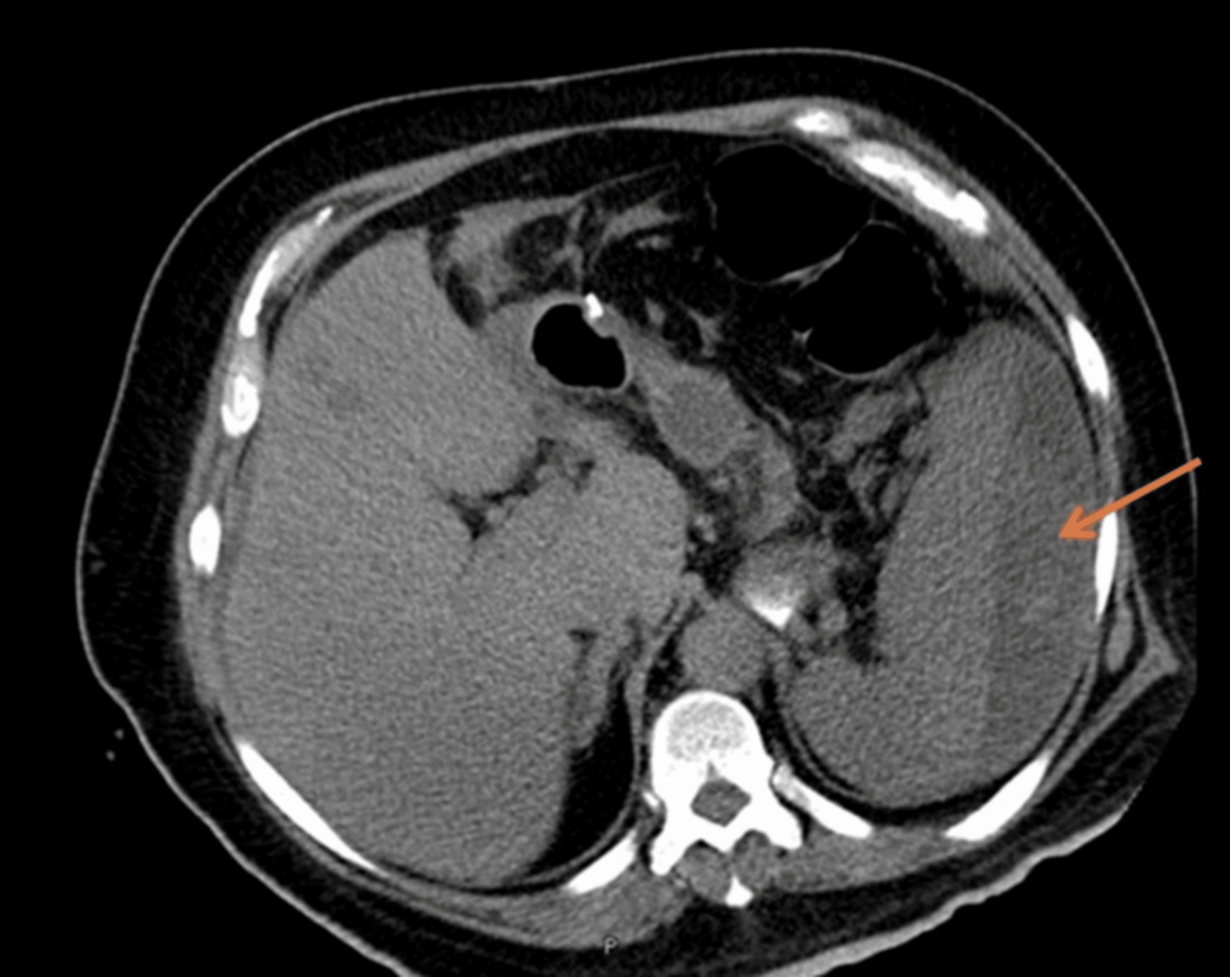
Non-contrast axial CT scan. The arrow is showing a hypodense area in the splenic region consistent with a perisplenic hematoma.
